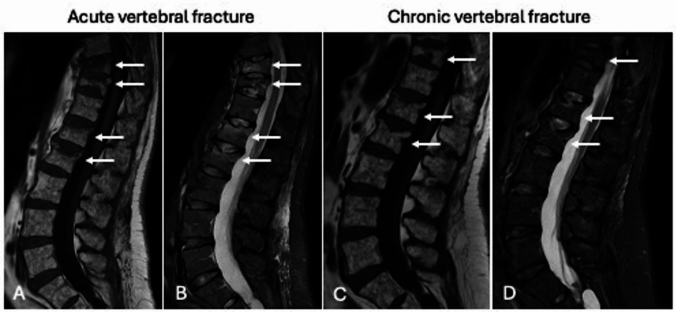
Vertebral fractures. MRI performed five days after trauma (A, B) show acute vertebral fractures (arrows) of T10, T11, L1, and L2, with bone edema appearing as bands along the collapsed endplates on STIR image (B) and associated with hypointense striae indicative of trabecular thickening on T1-weighted image (A). Spine MRI performed six months after trauma (T1-weighted on C, STIR image on D) shows chronic fractures (arrows) of T10, T11, L1, and L2, without bone edema
